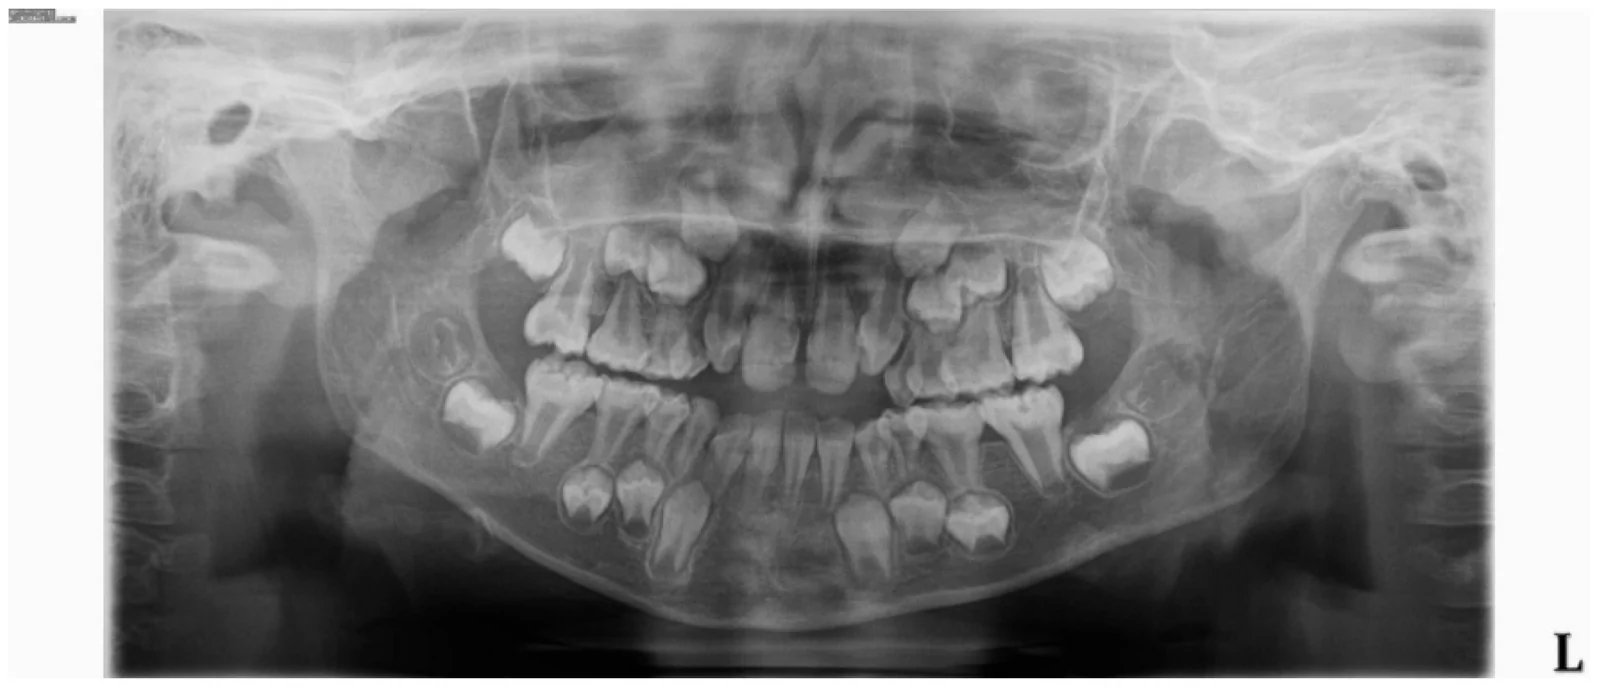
Panoramic radiograph of a 6-year-old girl included in the present study, demonstrating pyramidal molar morphology in both primary and permanent molars.
